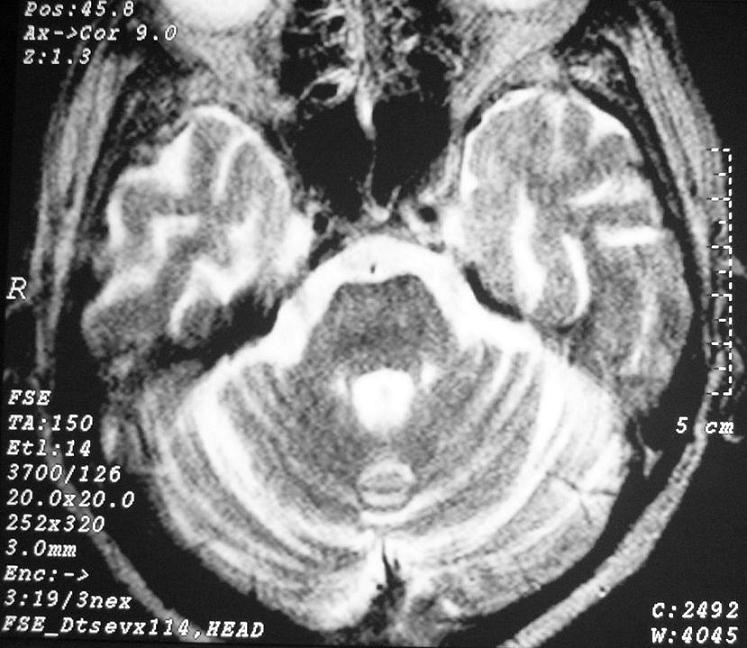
Brain axial T2W magnetic resonance imaging at the level of the pons. Widening of cerebellar sulci.
